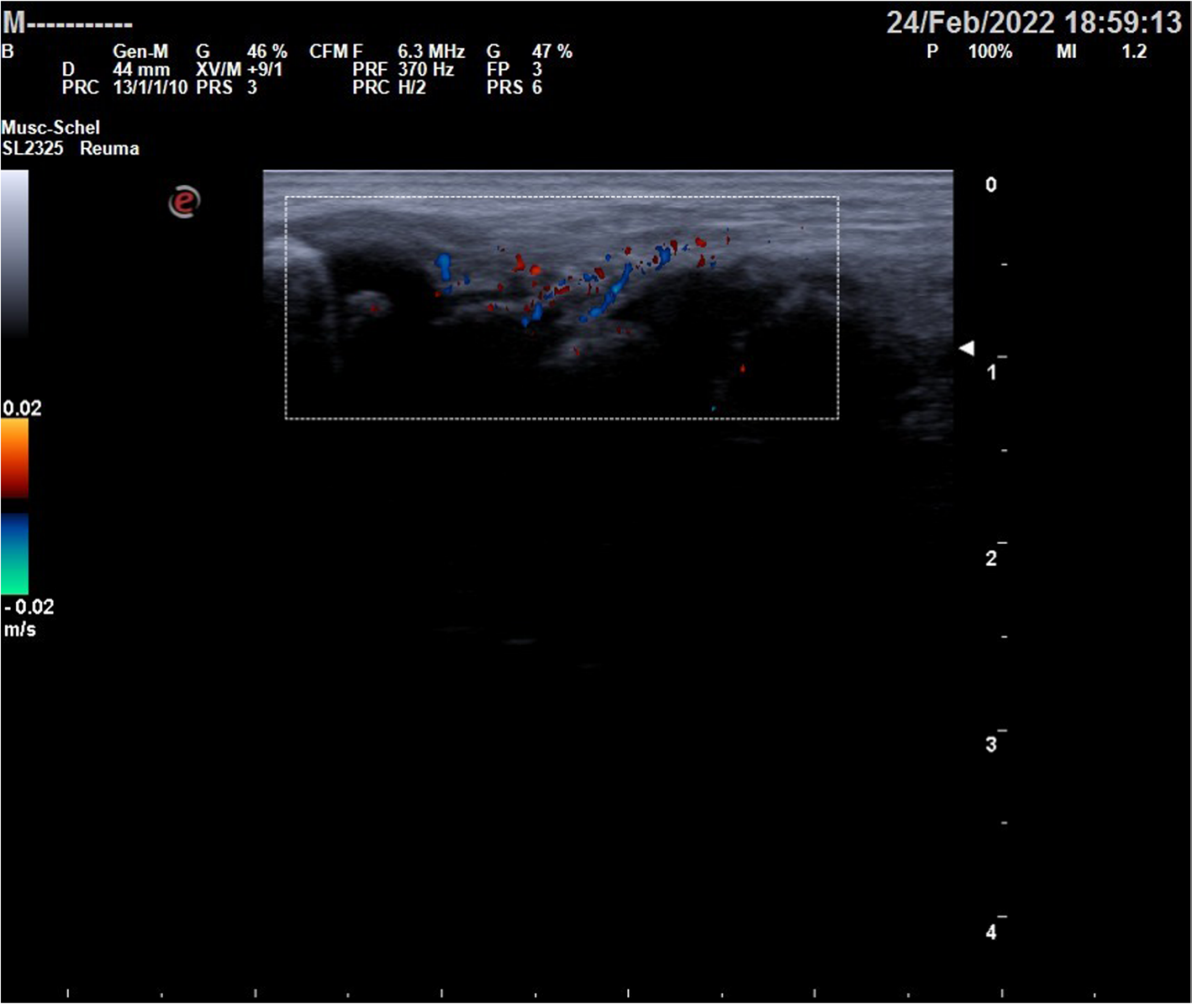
Wrists’ ultrasound shows synovial thickening with increased vascularity on colour Doppler
